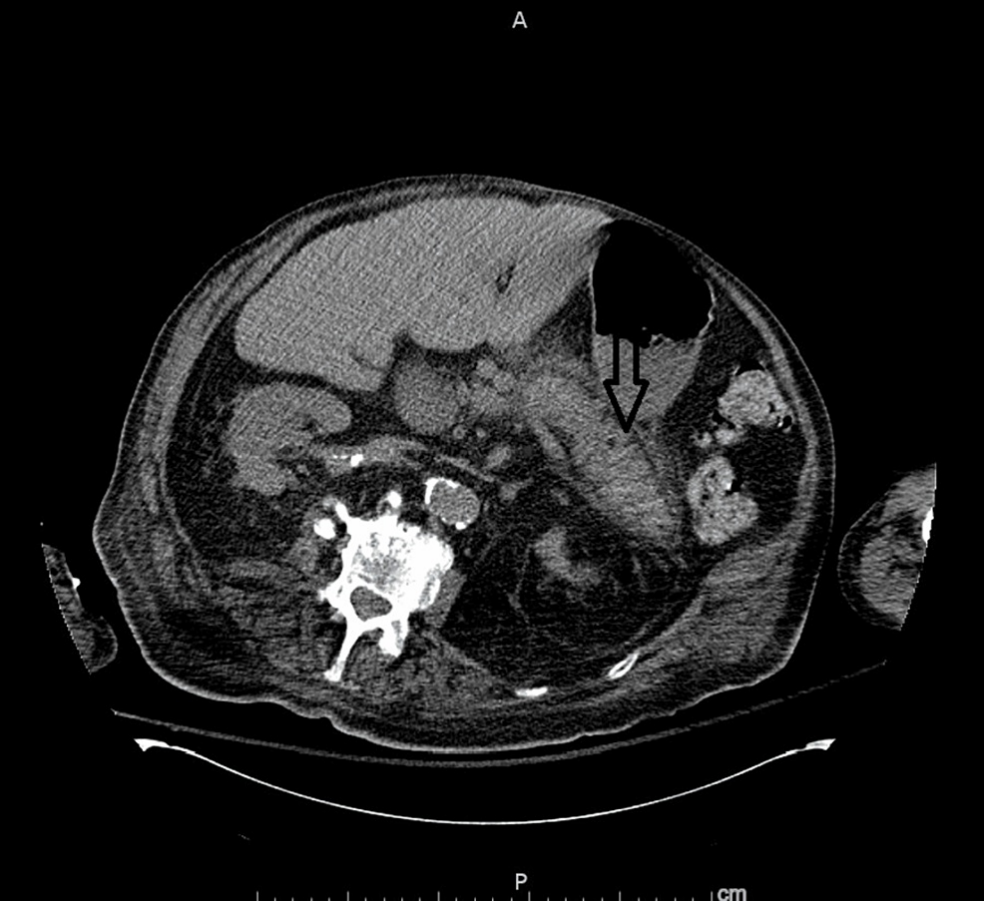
CT abdomen CT abdomen showing evidence of peripancreatic edema and stranding compatible with acute pancreatitis (arrow)
